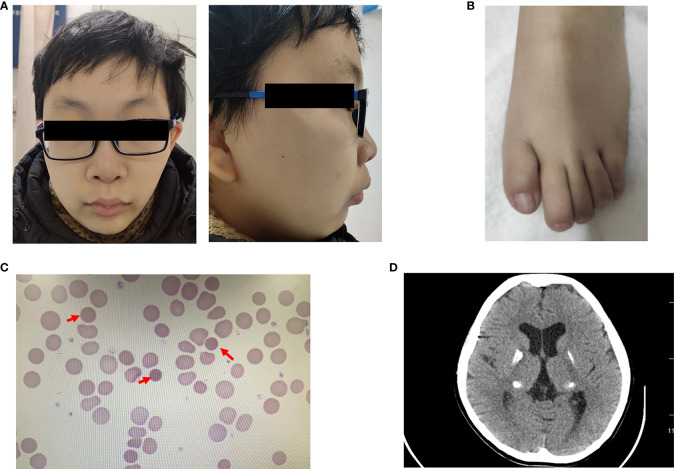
Clinical characters of the patient (A) Facial anomalies in the patient, including facial asymmetry, micrognathia, malformed ear, dental dysplasia, and a high-arched palate. (B) Feet anomalies showed broad and foreshortened halluces. (C) Peripheral blood smear revealed small spherocytes (red arrows). (D) Brain CT showed calcification in the bilateral lenticular nucleus, dorsal thalamus, and posterior horn of lateral ventricle.
